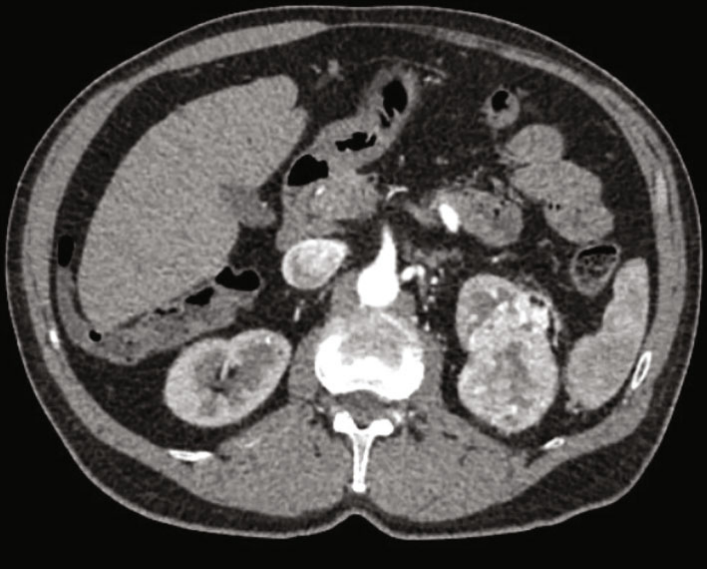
Axial CT scan showing posterior upper pole left enhancing renal mass concerning for malignancy.
